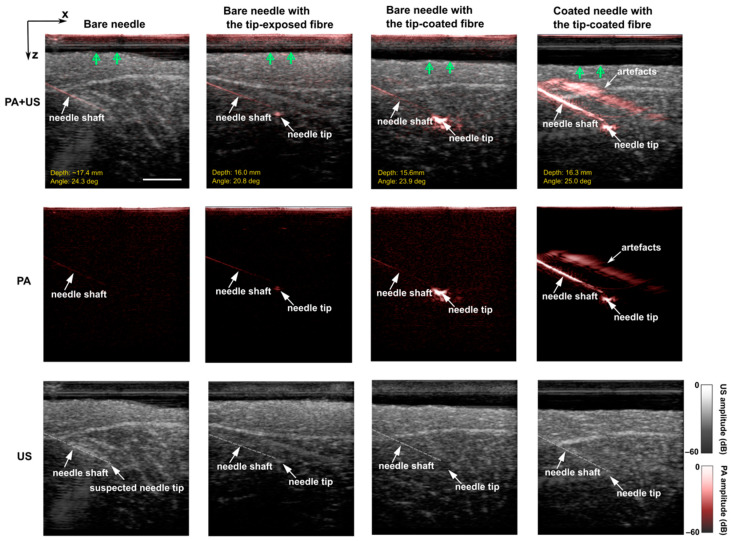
Overlaid Photoacoustic (PA) and Ultrasound (US) images, PA images, and US images acquired during in-plane needle insertions into a pork joint tissue ex-vivo with a bare needle, a bare needle with a tip-exposed fibre, a bare needle with a tip-coated fibre, and a coated needle with a tip-coated fibre. Insertion depth was defined as the shortest distance from the suspected needle tip to the skin surface (denoted by green arrows). Insertion angles were measured from the positive x-axis going clockwise. In US images, needles including the tip were marked (translucent white dashed lines) according to corresponding PA images. Images were obtained from real-time reconstructions and displayed on a logarithmic scale. All the images have the same scale bar of 1 cm.
